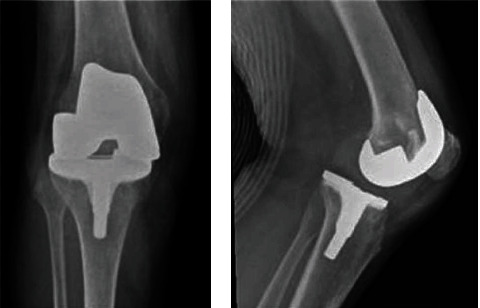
(a) Anteroposterior radiograph of the knee from case 1 at presentation in the emergency room. (b) Lateral radiograph of the knee from case 1 at presentation in the emergency room.
